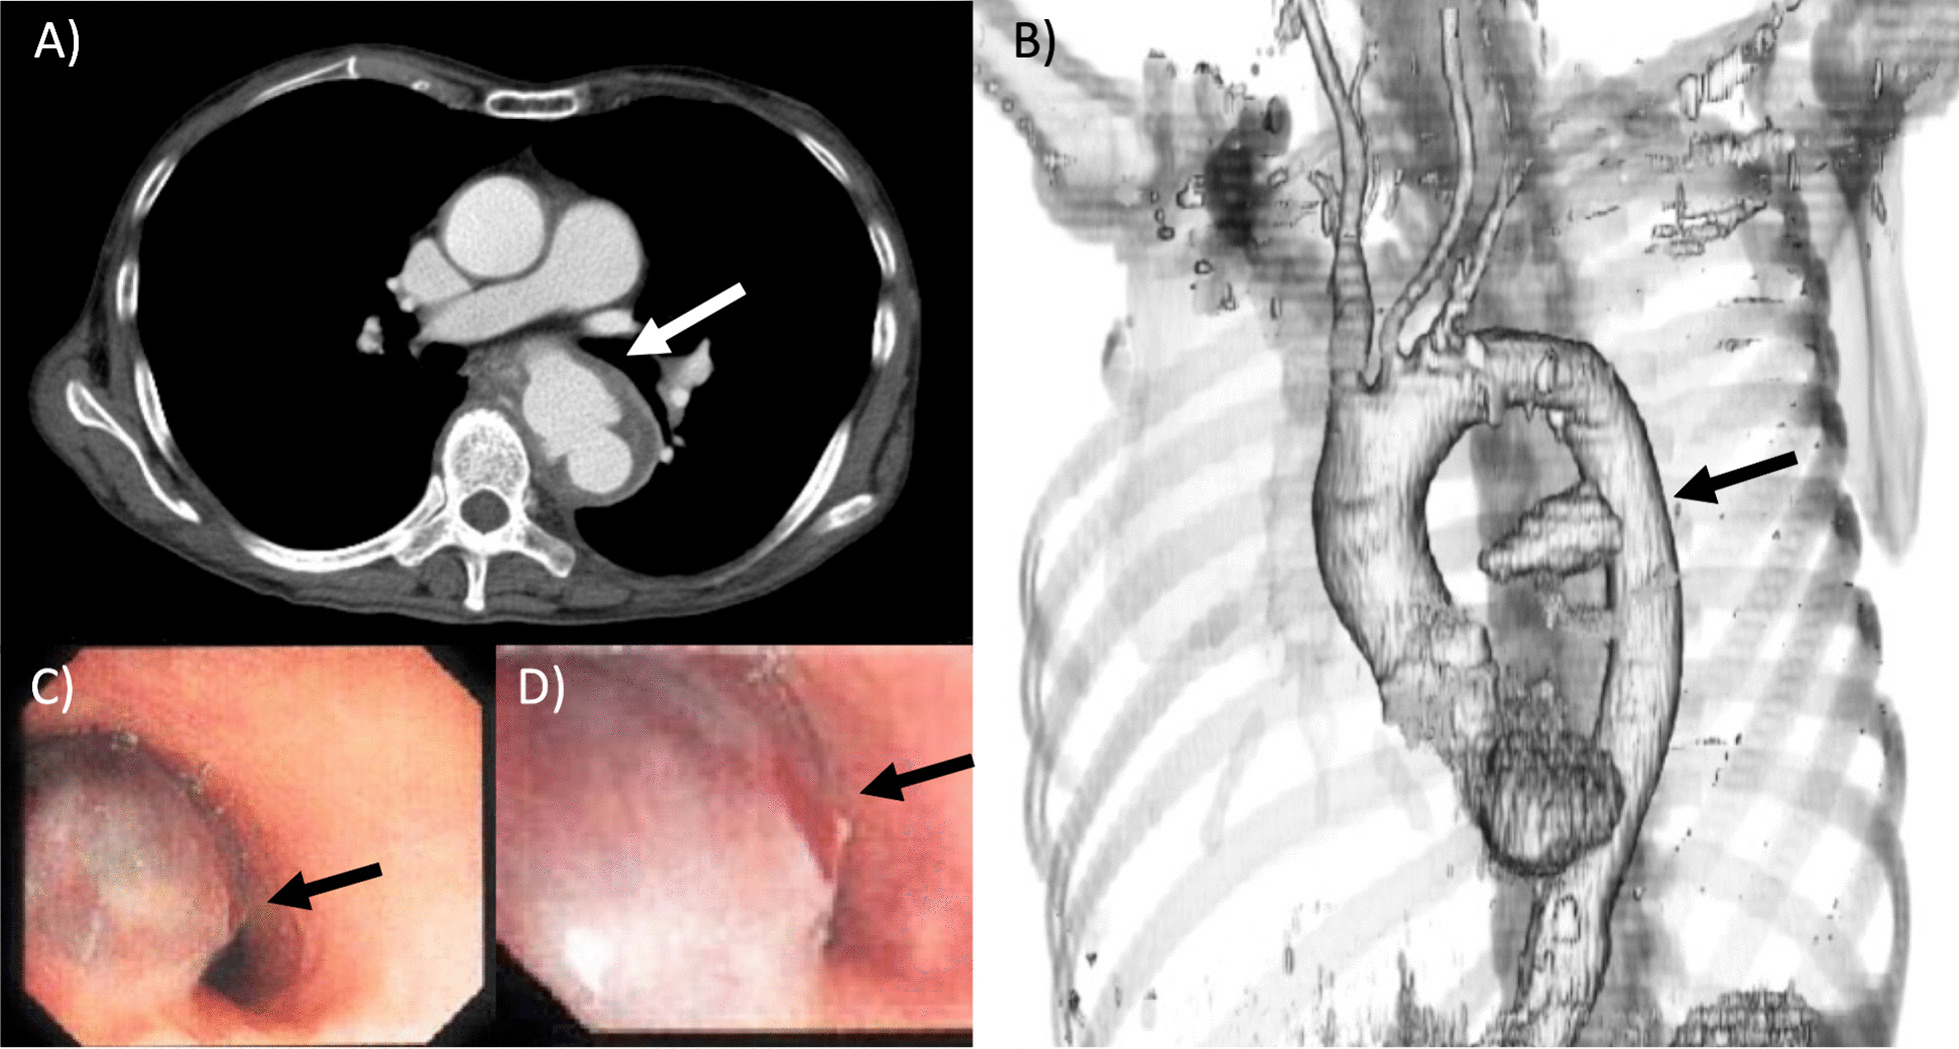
Preoperative computed tomography and endoscopy findings. a A computed tomography image of the rupture of a thoracic aortic aneurysm. The arrow indicates the position of the aneurysm. b A 3-dimensional computed tomography image. A saccular aneurysm protrudes into the descending aorta (arrow). c Upper gastrointestinal endoscopy. The arrow indicates aneurysm-related compression of the esophageal lumen. d Magnification of the image in (c). Site of perforation and blood points observed from the esophageal lumen (arrow)
